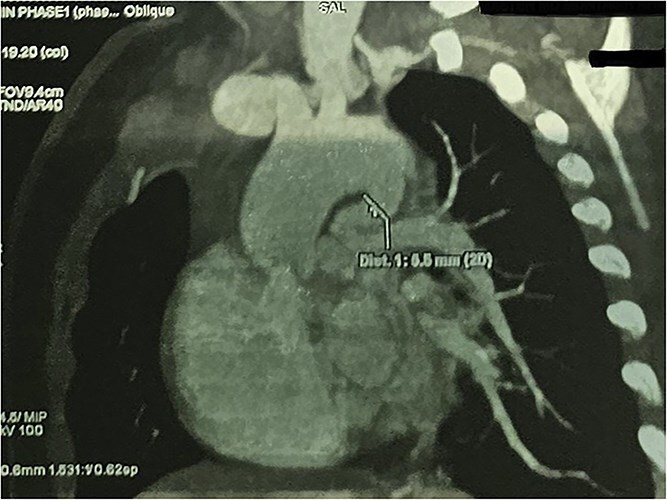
CTA demonstrating max PDA caliber with focal narrowing near LPA insertion.
